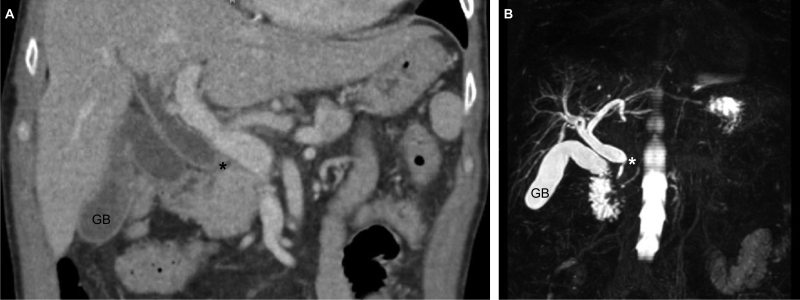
CT (A) and MRCP (B) images show a markedly dilated biliary tree above the surgical choledochoduodenostomy (*asterisks*). CT, computed tomography; MRCP, magnetic resonance cholangiopancreatography; GB, gallbladder.
